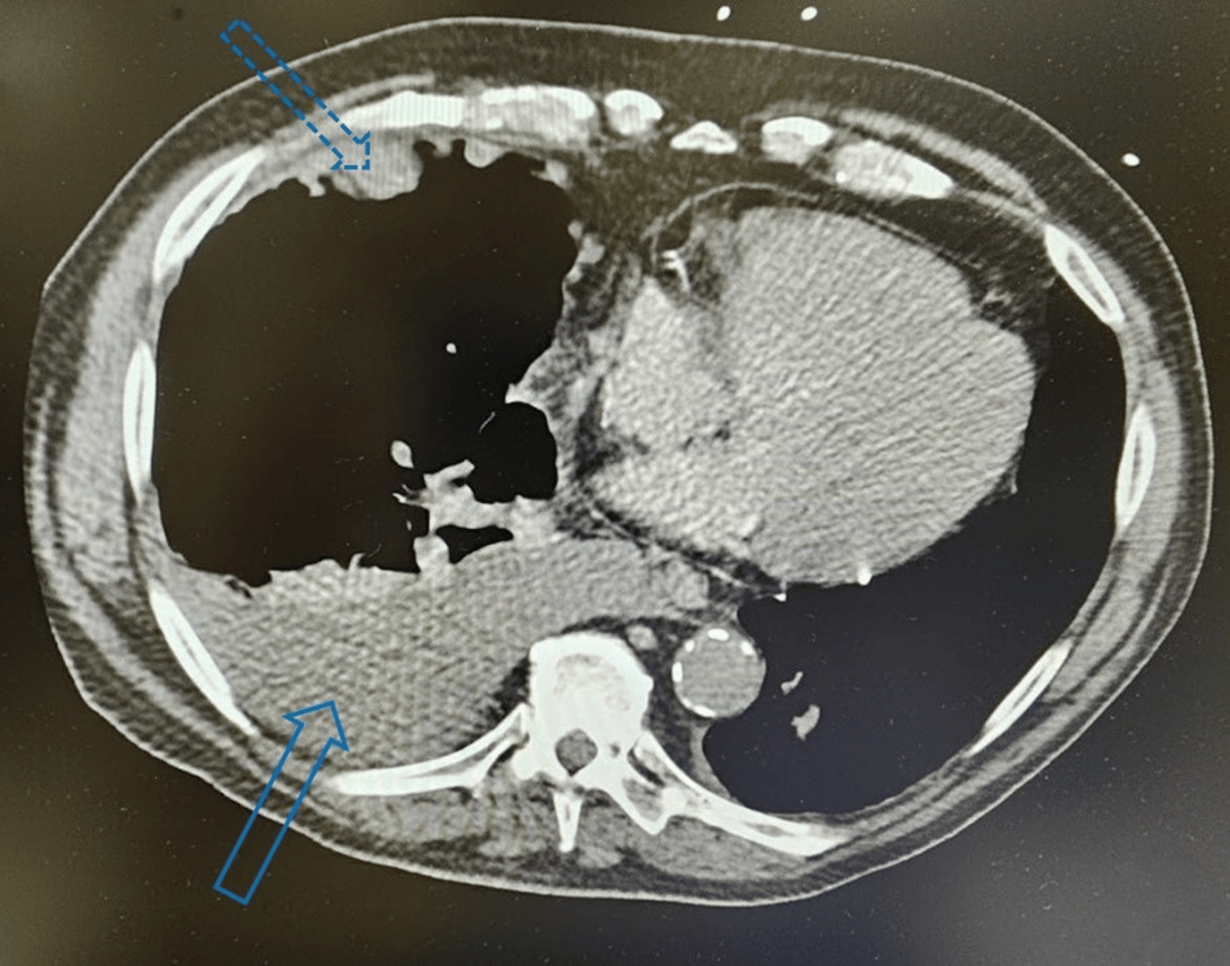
CT scan showing right pleural effusion The solid blue arrow at the bottom of the screen shows a significant pleural effusion in the right pleural space. The dotted blue arrow at the top of the screen displays peripheral nodularity CT: computed tomography
